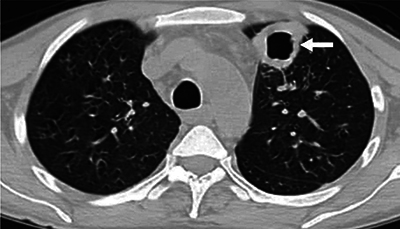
Non-contrast CT axial sections show a subpleural thick-walled cavitary lesion in the anterior segment of the upper lobe of the left lung (white arrow).
